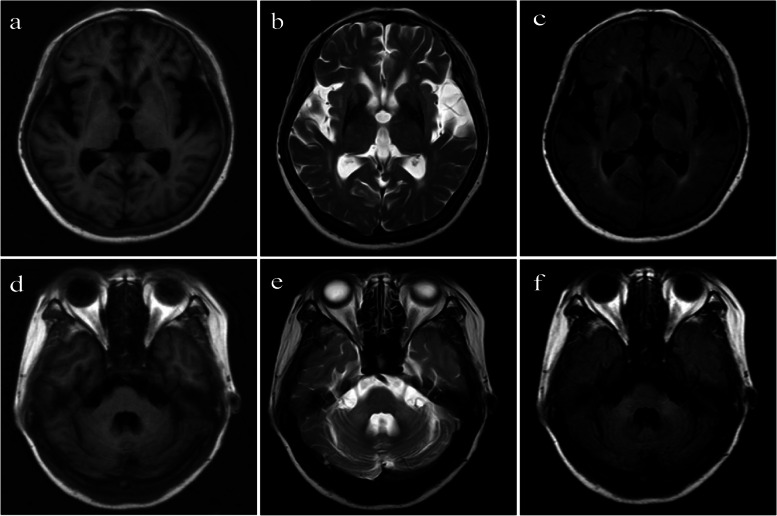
MRI showed atrophy of the brainstem and cerebellum, enlarged bilateral ventricle, iron deposition in the bilateral putamen, and a hyperintense putaminal rim. a and d Contrast-enhanced T1-weighted image. b and e Contrast-enhanced T2-weighted image. c and f T2/FLAIR image. MRI: Magnetic resonance imaging; FLAIR: Fluid attenuation inversion recovery
